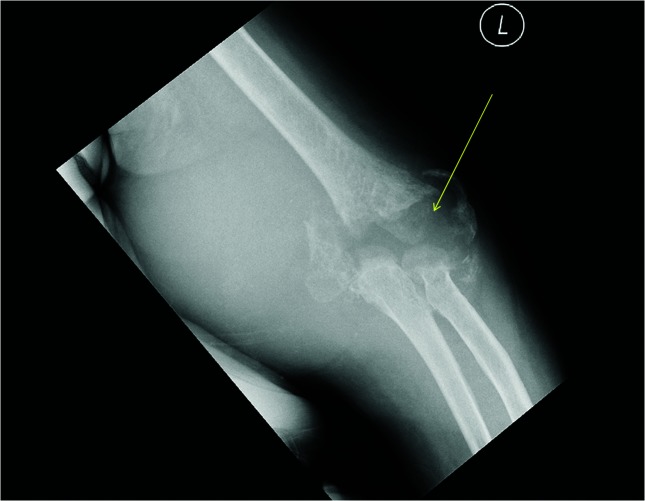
X-ray of the left elbow joint shows osteomyelitis and destruction of the joint as indicated by the arrow.
